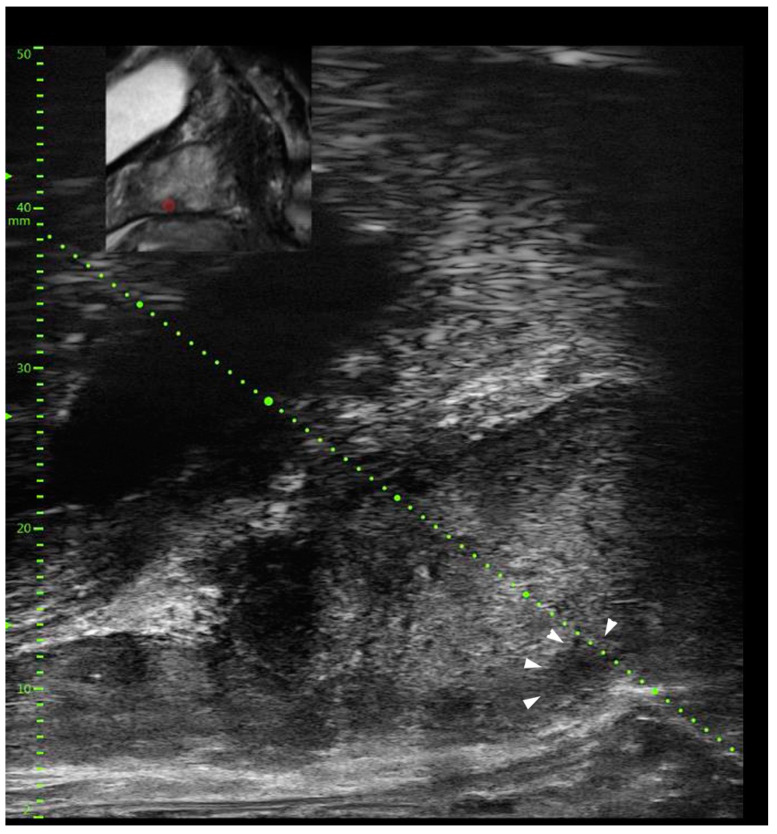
MRI lesion (red dot on MRI image in the upper left corner) is clearly visualized in real-time on microUS (outlined by the arrowheads) and provides the operator the confidence that the lesion found on MRI is the same as seen on microUS. Additionally, mpMRI/microUS fusion is beneficial in targeting MRI suspicious sites in the anterior gland, which may not be visible on microUS in real-time for biopsy.
